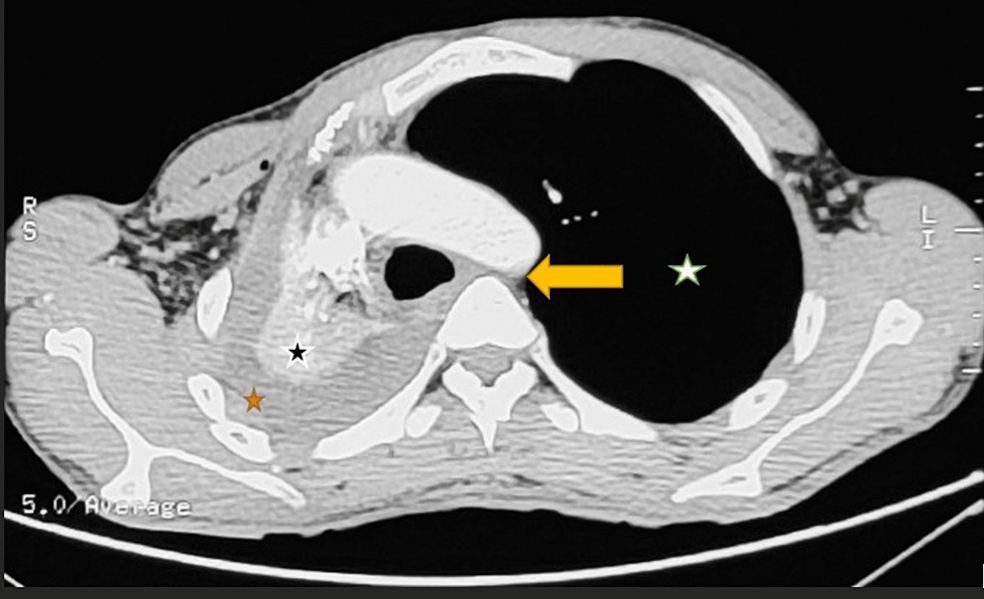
CT chest without contrast (transverse section) at the level of the mid thorax The image shows a collapsed right lung (orange star) along with moderate hemothorax (black star). It also shows a mediastinal shift and tracheal shift (yellow arrow) to the right and a compensatory over-expansion of the left lung (white star) CT: computed tomography
